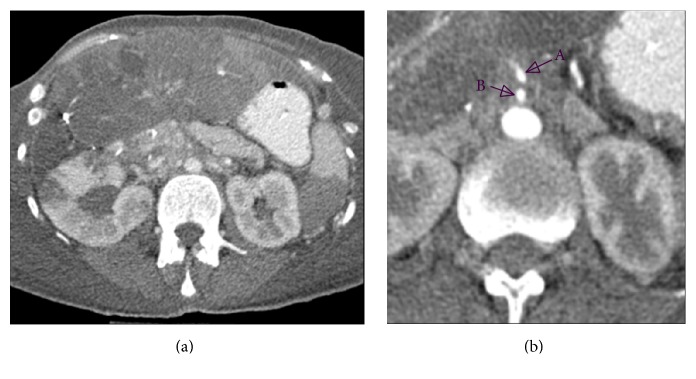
Abdominal CT-scan. (a) Centralized perfusion with constricted peripheral intestinal arteries. (b) Reduced diameters of abdominal arteries. (A) Truncus coeliacus: 3 mm. (B) A. mesenterica sup.: 3 mm; aorta 15 mm.
